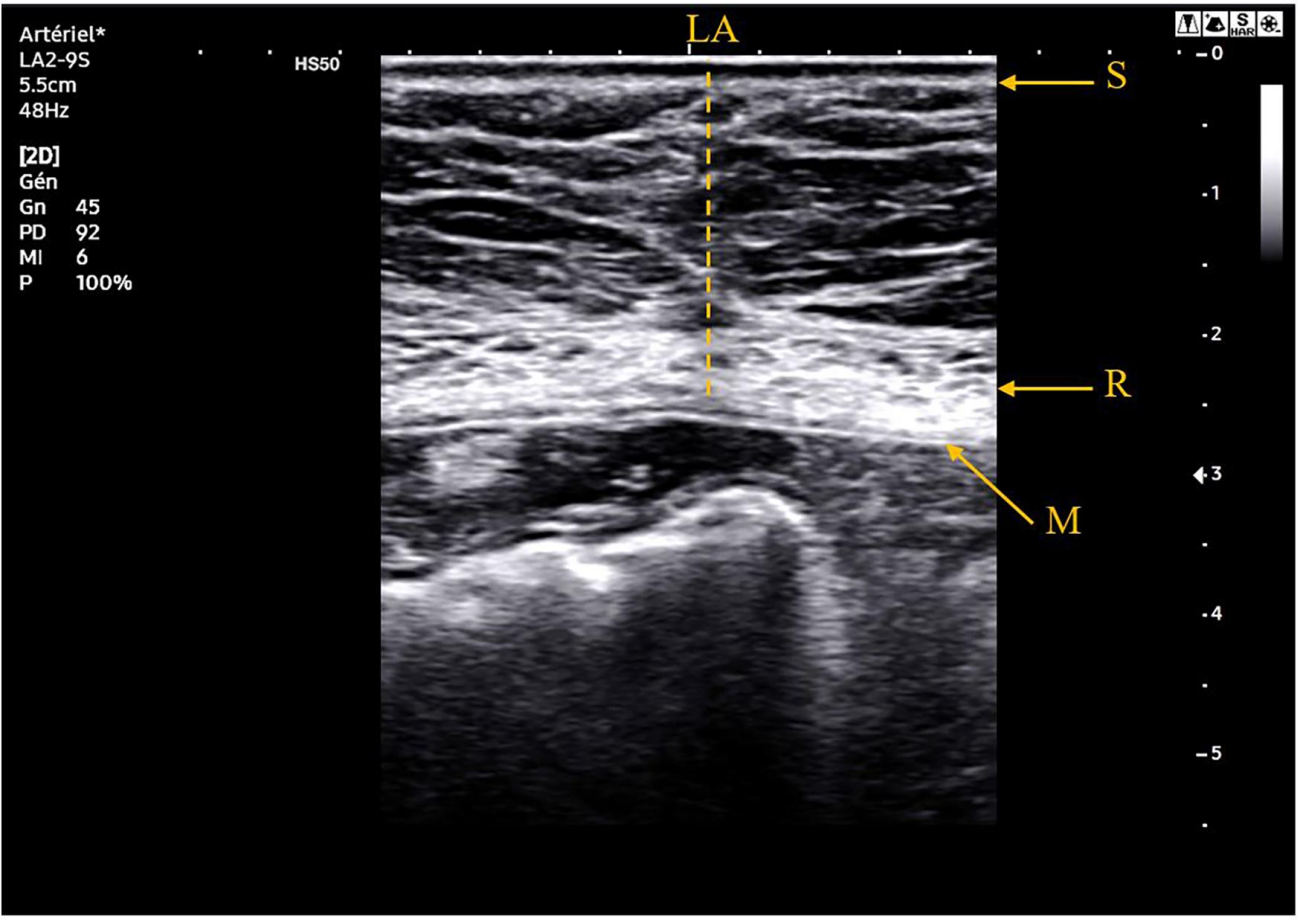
Ultrasound image at 78 months of follow-up of a 76-year-old patient who did not present any incisional hernia. S, skin; R, rectus abdomini muscle; LA, linea alba; M, retromuscular mesh.
